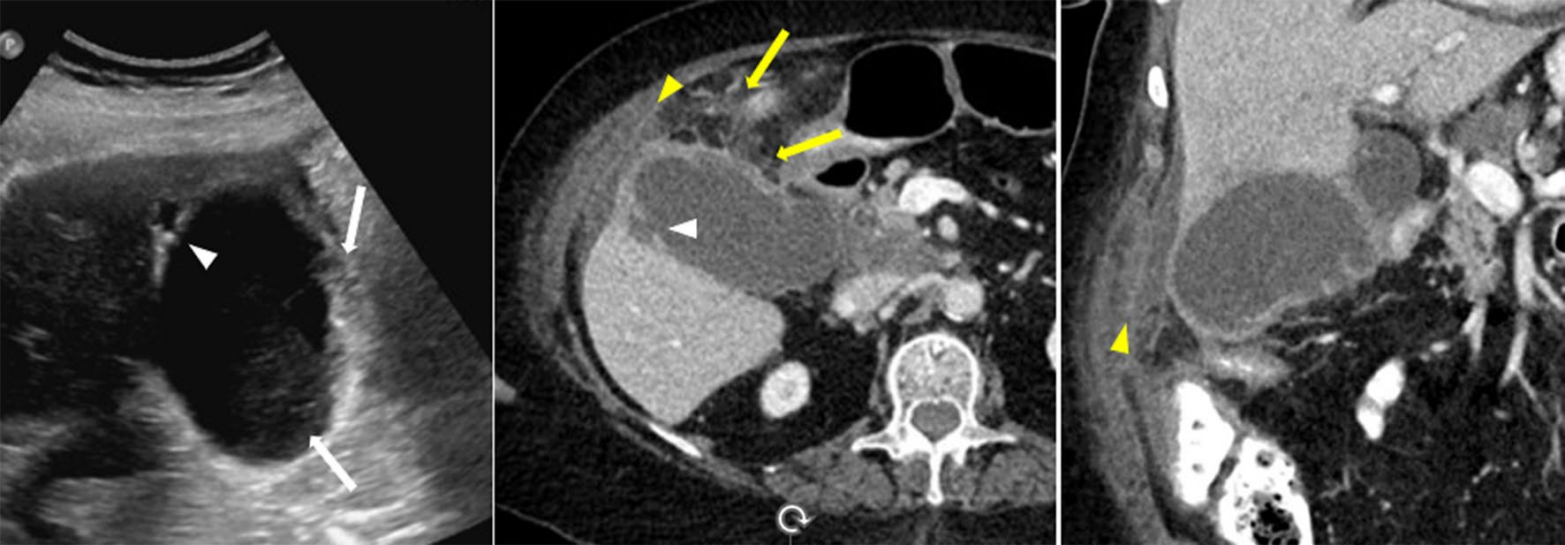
Gangrenous cholecystitis with perforation and peritonitis in a 91-year-old female with history of hypertension, presents with two weeks of right hemi-abdominal pain to the emergency room. Blood work showed, elevated white blood cell count, and elevation of the alkaline phosphatase. US was performed which demonstrates defect in the wall (arrowhead) in addition to the sludge and sloughed mucosa (arrows). Contrast enhanced CT confirms the finding of perforation (arrowhead). There is pericholecystic stranding (yellow arrow) and enhancement of the peritoneal lining secondary to peritonitis (yellow arrowhead) resulting from bile leak
